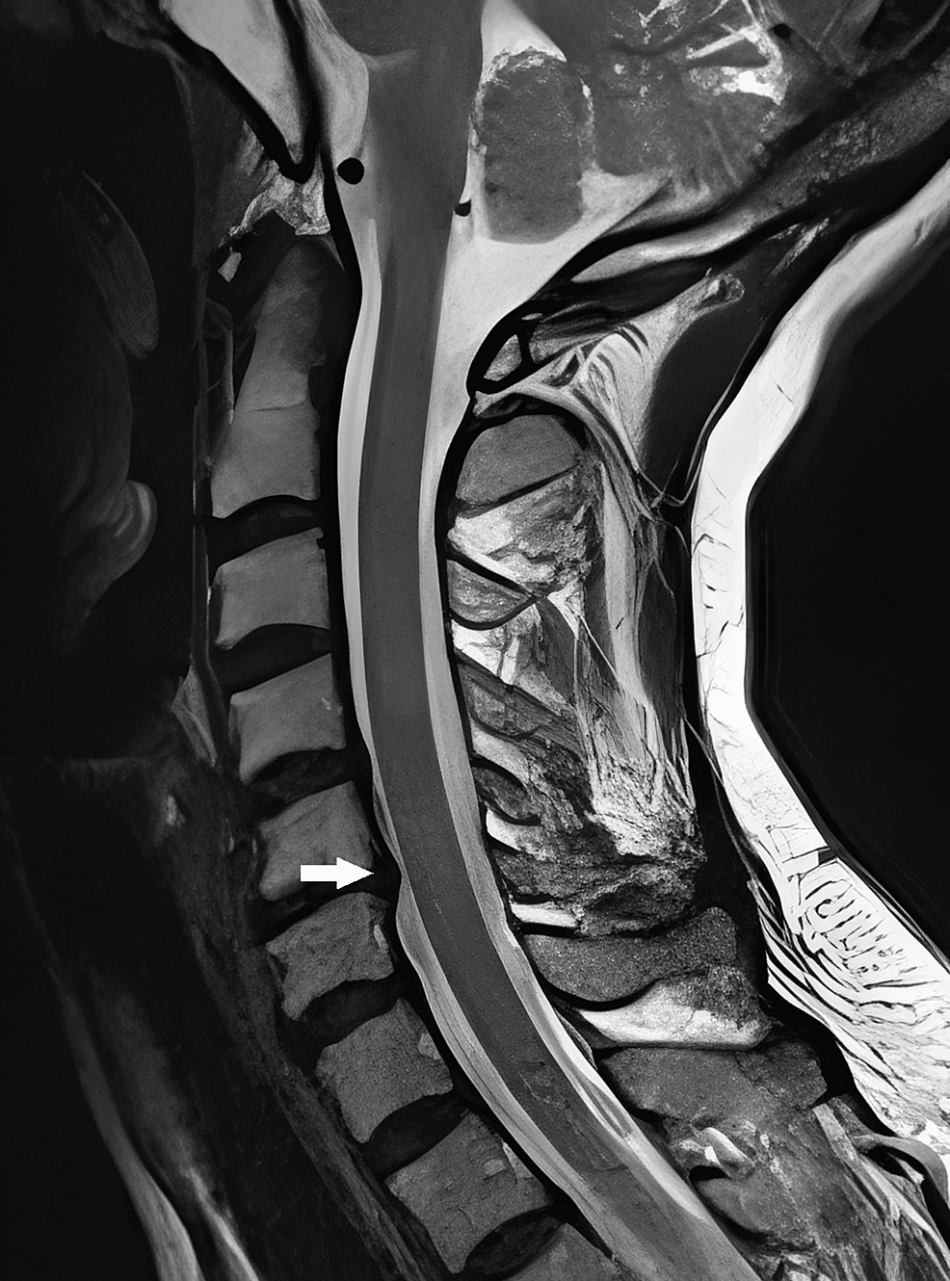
Sagittal T2-weighted MRI demonstrating a disc herniation at the C5-6 level (white arrow).
